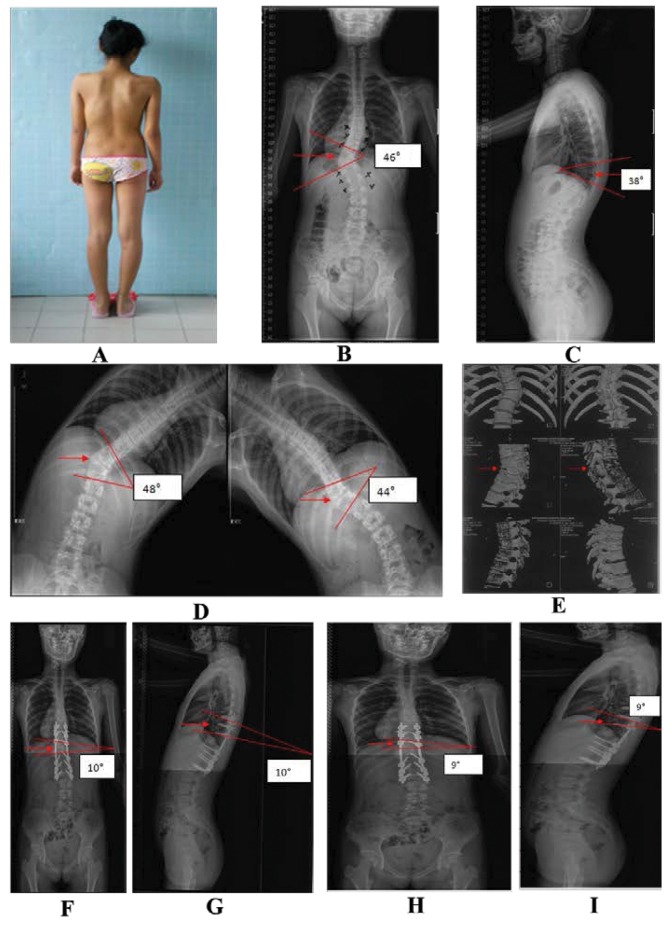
Images of a 12-year-old girl who underwent hemivertebra resection and monosegmental fusion for T11 hemivertebra deformity: Preoperative gross inspection (A); Preoperative anteroposterior x-ray indicating T11 hemivertebra deformity (arrow) with the scoliosis convex to the left and concave to the right, and a scoliosis Cobb angle of 46º (B); Preoperative lateral x-ray indicating T11 hemivertebra deformity (arrow) with a kyphosis Cobb angle of 38º (C); Preoperative left and right bending x-rays indicating T11 hemivertebra deformity (arrow) with a kyphosis Cobb angle of 48º and 44º (D); Preoperative computed tomography indicating T11 hemivertebra deformity (arrow) (E); Postoperative anteroposterior x-ray indicating the T11 hemivertebra had been resected (arrow) and good correction of the spine deformity had been achieved with a scoliosis Cobb angle of 10º (F); Postoperative lateral x-ray indicating the T11 hemivertebra had been resected (arrow) and good correction of the spine deformity had been achieved with a kyphosis Cobb angle of 10º (G); Anteroposterior x-ray 3 years following surgery indicating the T11 hemivertebra had been resected (arrow) with a scoliosis Cobb angle of 9º (H); Lateral x-ray 3 years following surgery indicating the T11 hemivertebra had been resected (arrow) with a kyphosis Cobb angle of 9º (I).
